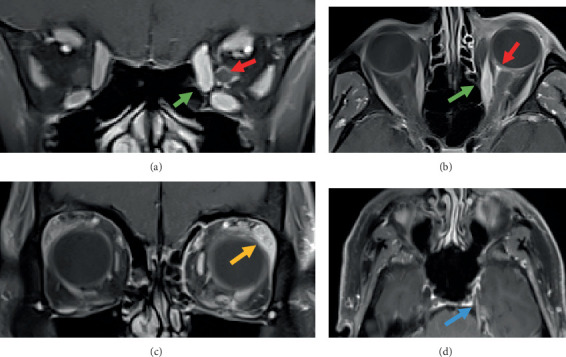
Fat-suppressed contrast-enhanced MRI demonstrating (a, b) enlargement and enhancement of the left medial rectus, inferior rectus and superior oblique muscles (green arrows), (a, b) enhancement of the left optic nerve sheath (red arrows), (c) lacrimal gland enhancement and enlargement (orange arrow) and (d) enhancement of the prepontine left trigeminal nerve (blue arrow).
